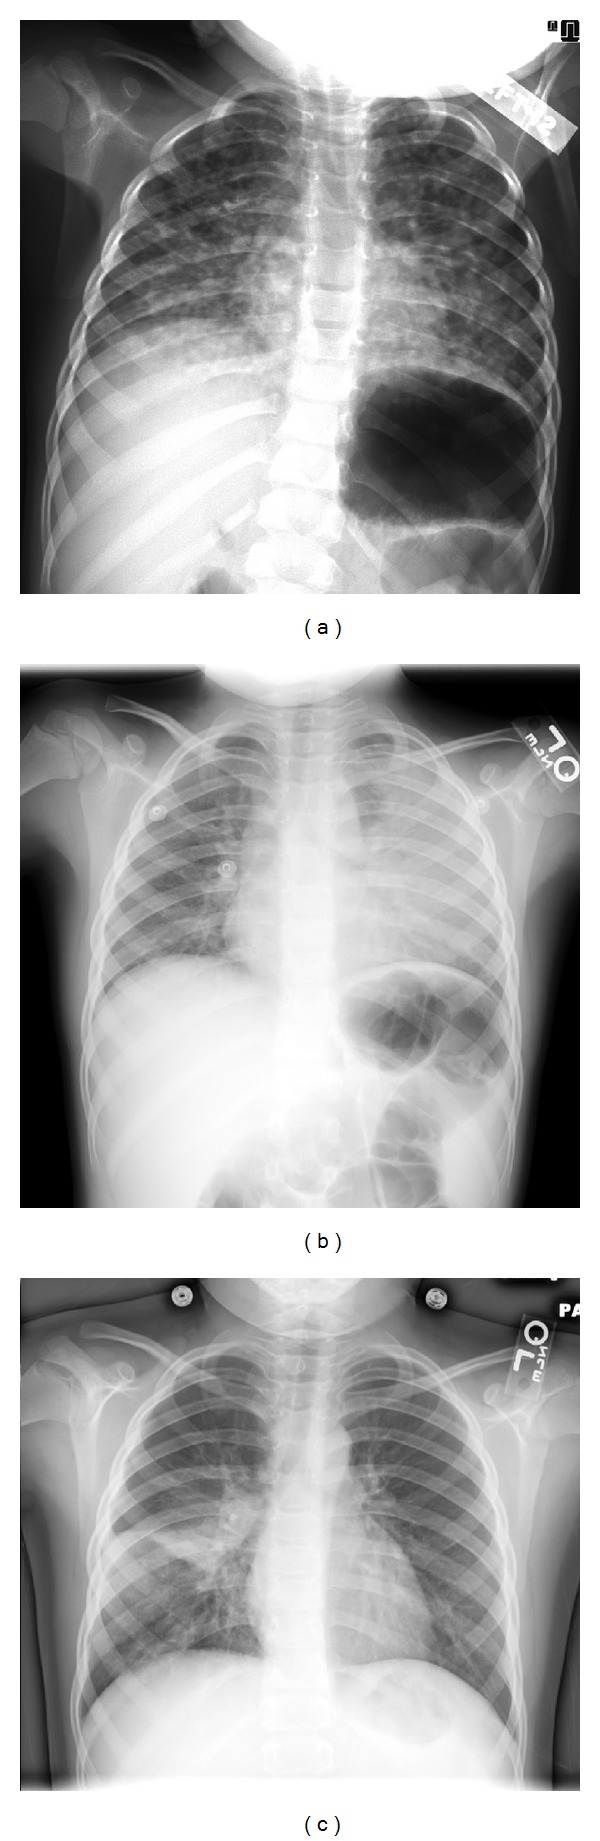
Chest radiographs prior to admission. Images demonstrating focal opacities with variable patterns, from extensive diffuse nodularity (a) to large opacifications involving several areas of the lungs ((b) and (c)). These films were taken at 3 years (a), 6 years (b), and 8 years (c) of age during prior episodes of pneumonia.
